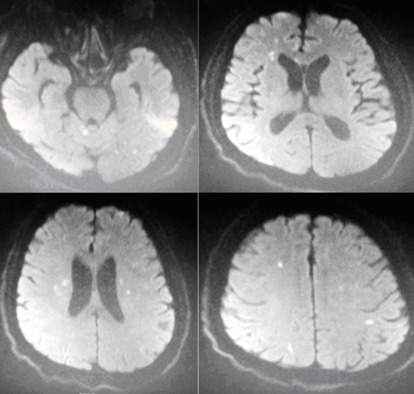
Multicentric acute ischemic hyperdensity of the brain diffusion MRI
